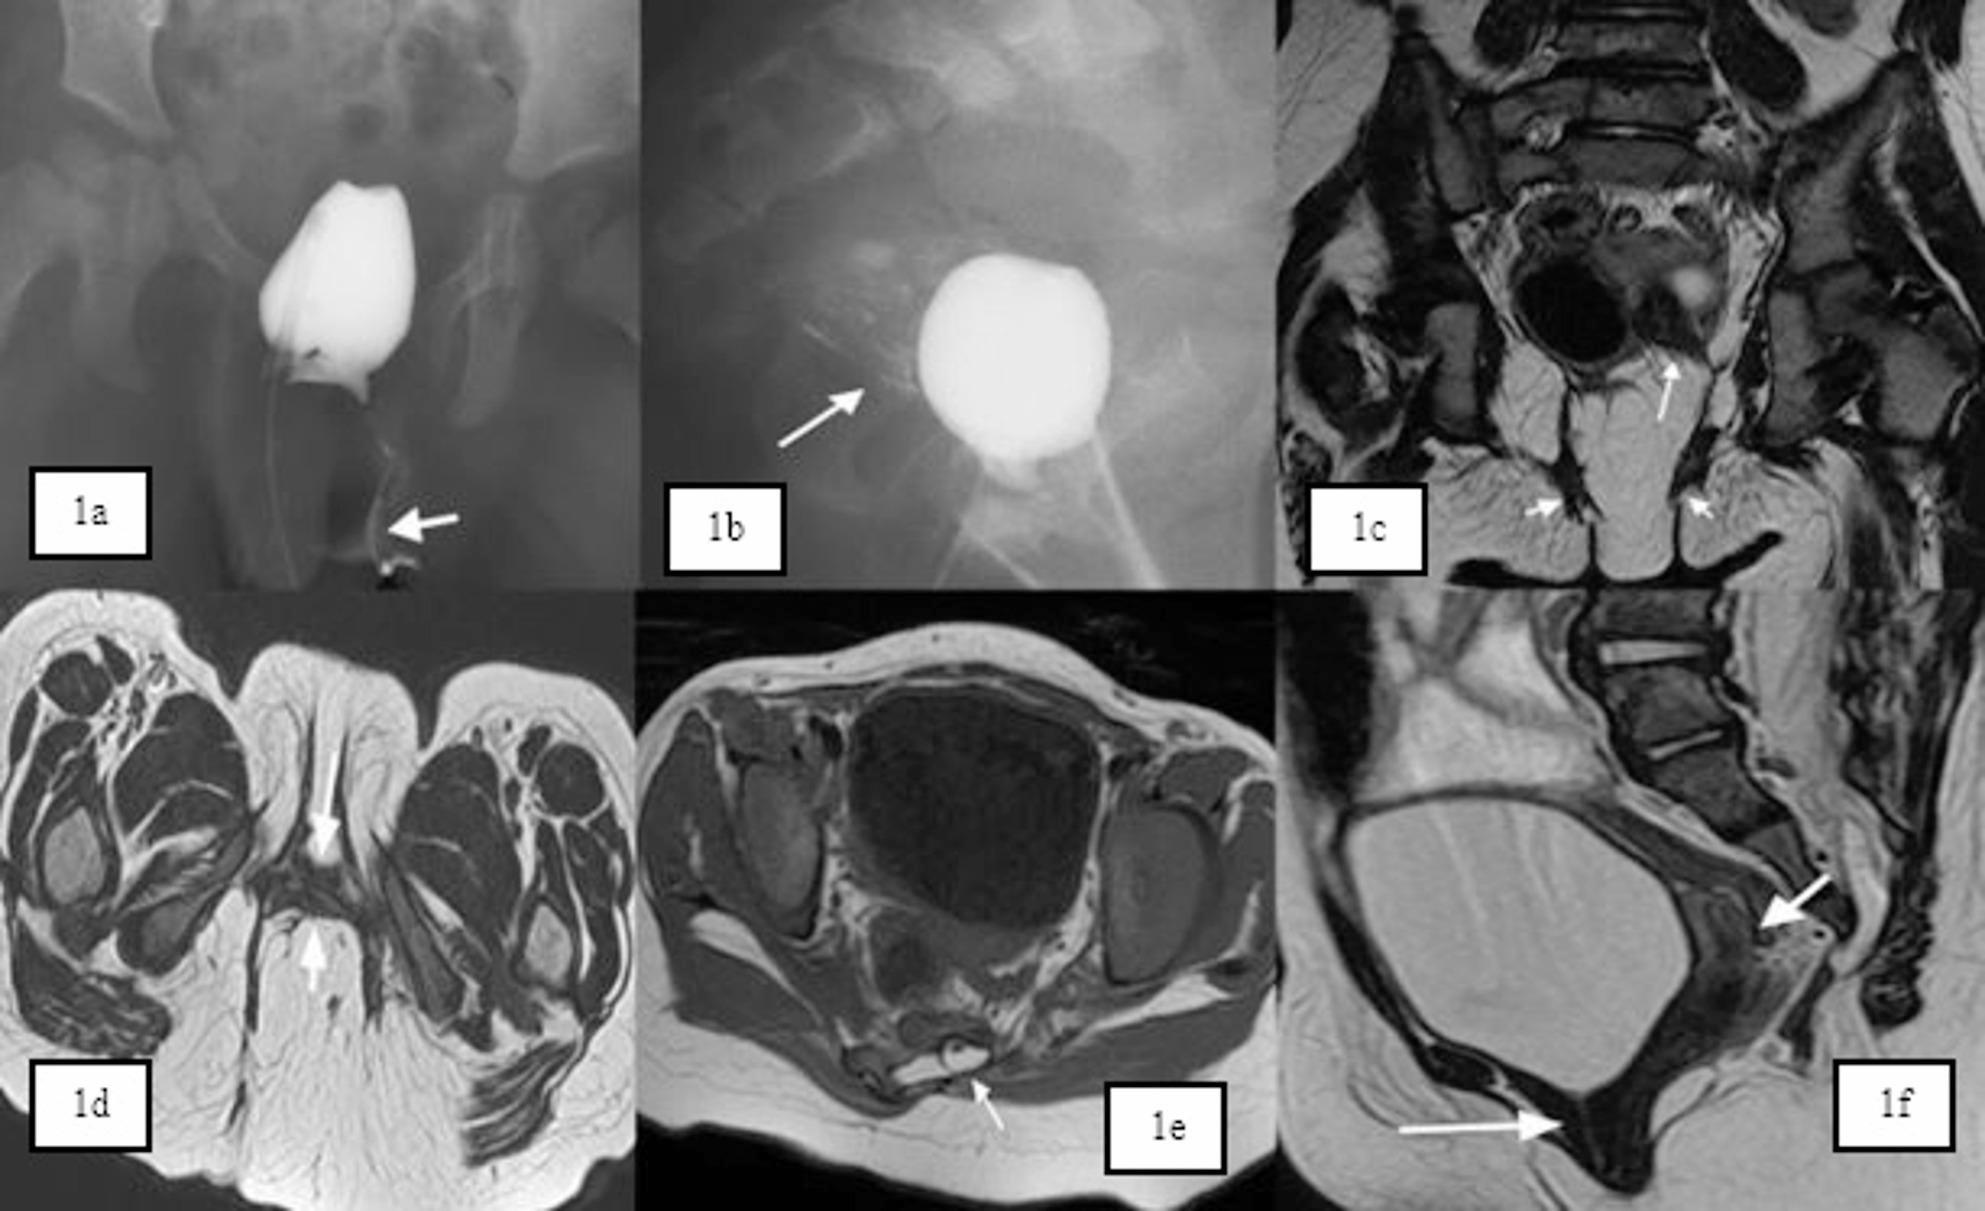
a & b: Genitography examination and opacification of vagina and uterus. c - f: MRI pelvis
